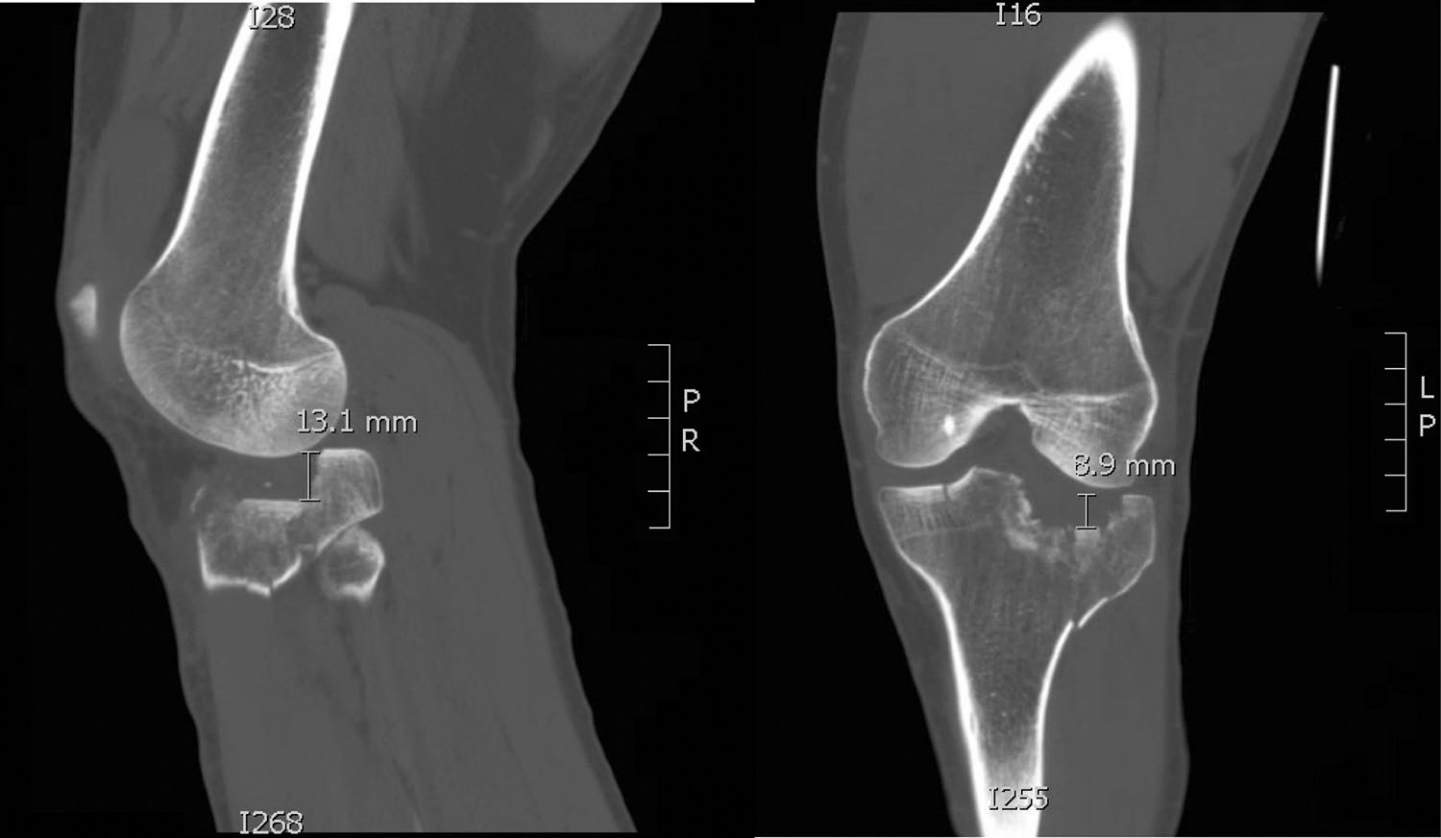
Sagittal and Coronal CT of the knee demonstrating articular depression measured on both views with 13.1 mm of depression on the sagittal, 8.9 mm depression on coronal imaging
